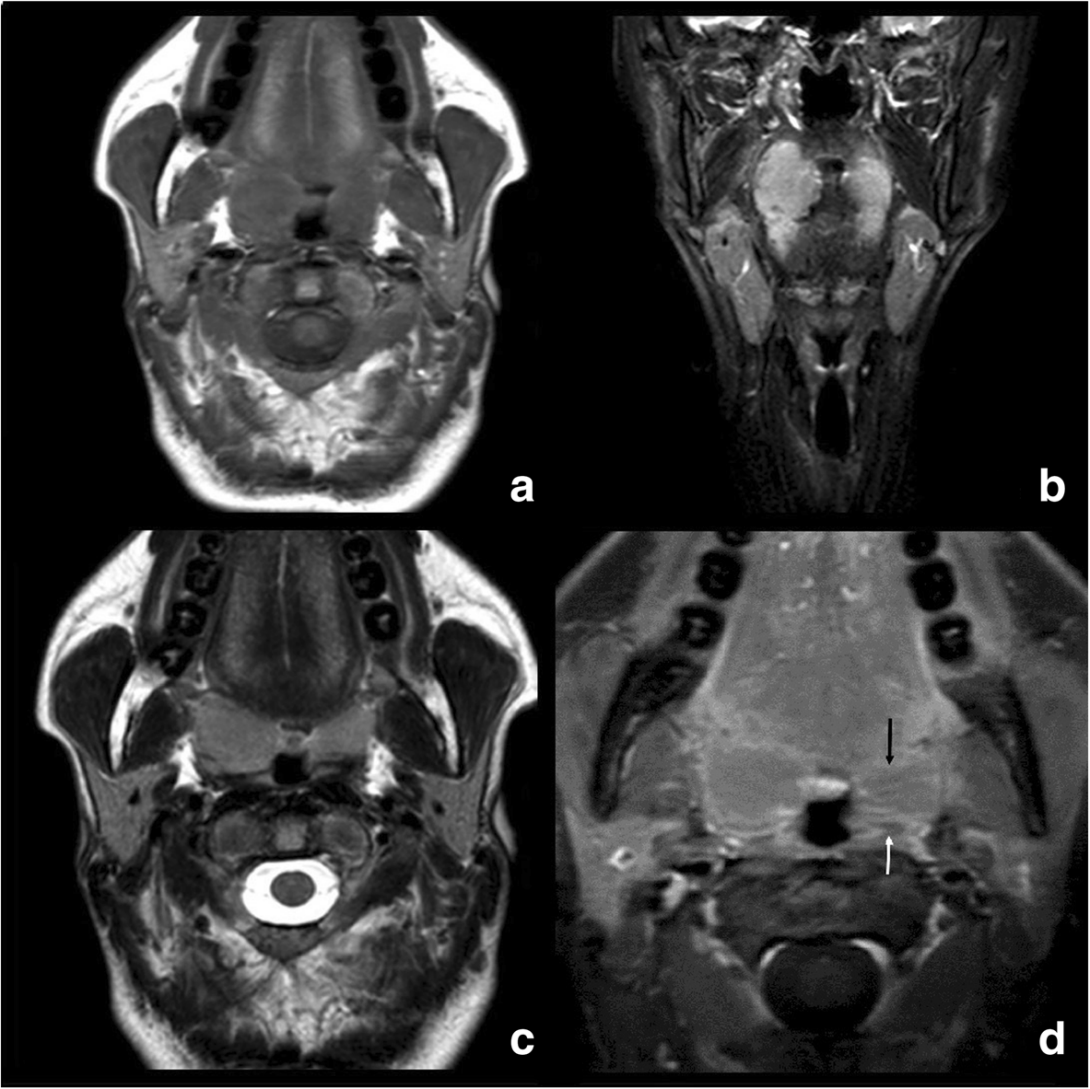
Axial noncontrast T1 (a), coronal STIR (b), Axial T2-weighted (c) and postgadolinium T1-weighted with fat saturation (d) MR images. Note the obliteration of the right palatine tonsillar crypts combined with linear horizontal enhancement to the left (arrow in d)
